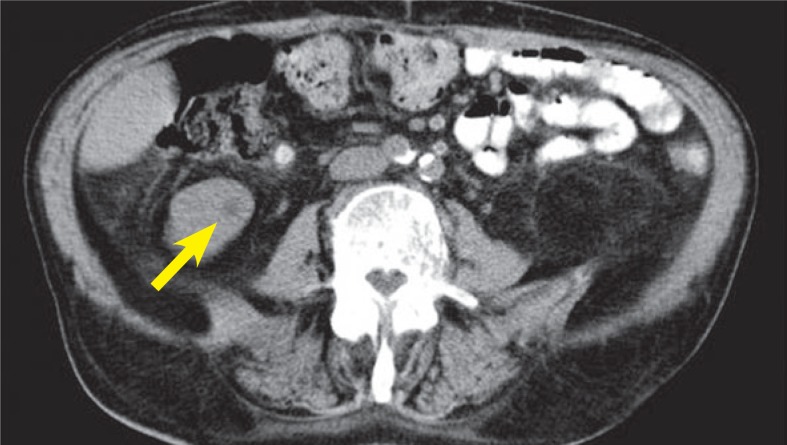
Abdominal CT scan shows small primary renal tumor in the the right kidney (contrast enchanced CT was not performed due to patient’s poor renal function with glomerular filtration less then 30 mL/min/1.73 m^2^).
